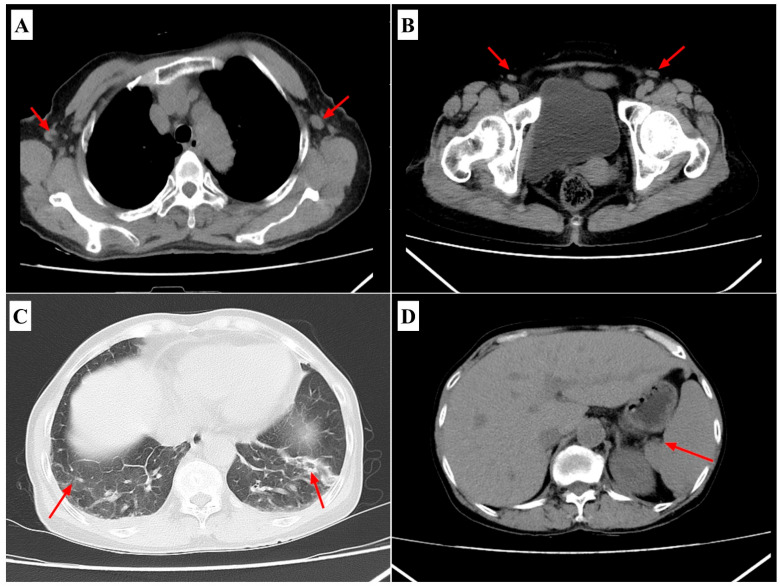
CT scan of the chest demonstrates. (A) Axillary lymphadenopathy; (B) inguinal lymphadenopathy; (C) bilateral multiple pulmonary inflammatory lesions, predominantly in the lower lobes; (D) splenomegaly. (The red arrow in the figure indicates the lesion site).
